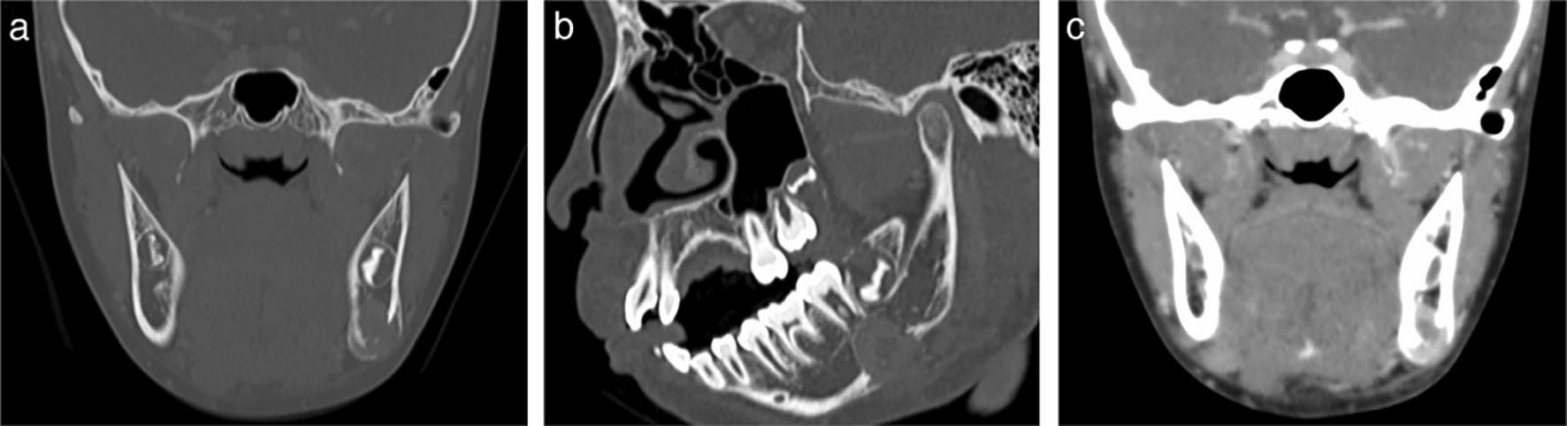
Computed tomography (CT) imaging of the lesion in the left mandible. CT showing an exophytic juxtacortical mass eroding the cortical bone of the left mandible with bone window settings (window width: 3000 HU; window level: 600 HU). Note the periosteal reaction outside the lesion. a Coronal image. b Sagittal image. c Coronal contrast-enhanced image showing weak contrast enhancement inside the lesion
